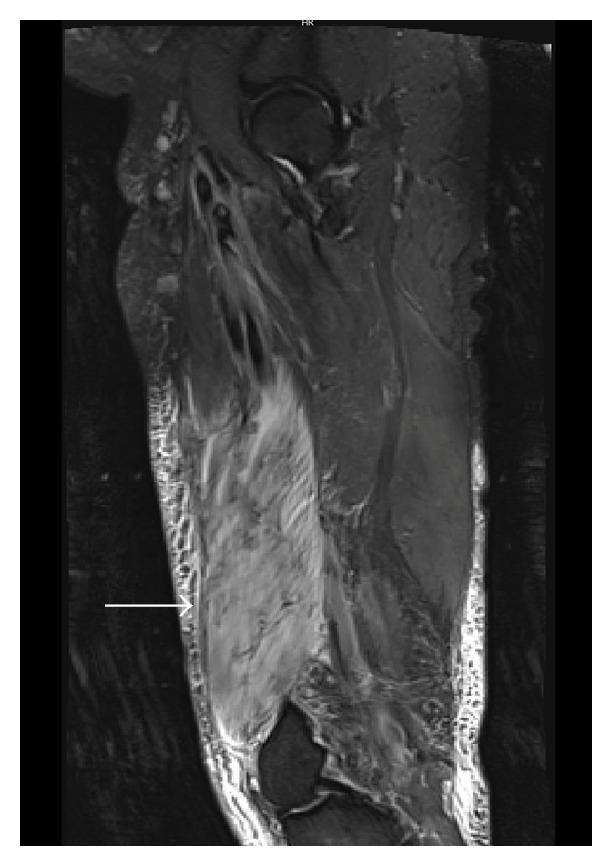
MRI of the left thigh, sagittal section, showing hyperintense signals on T2 weighted images (white arrow).
